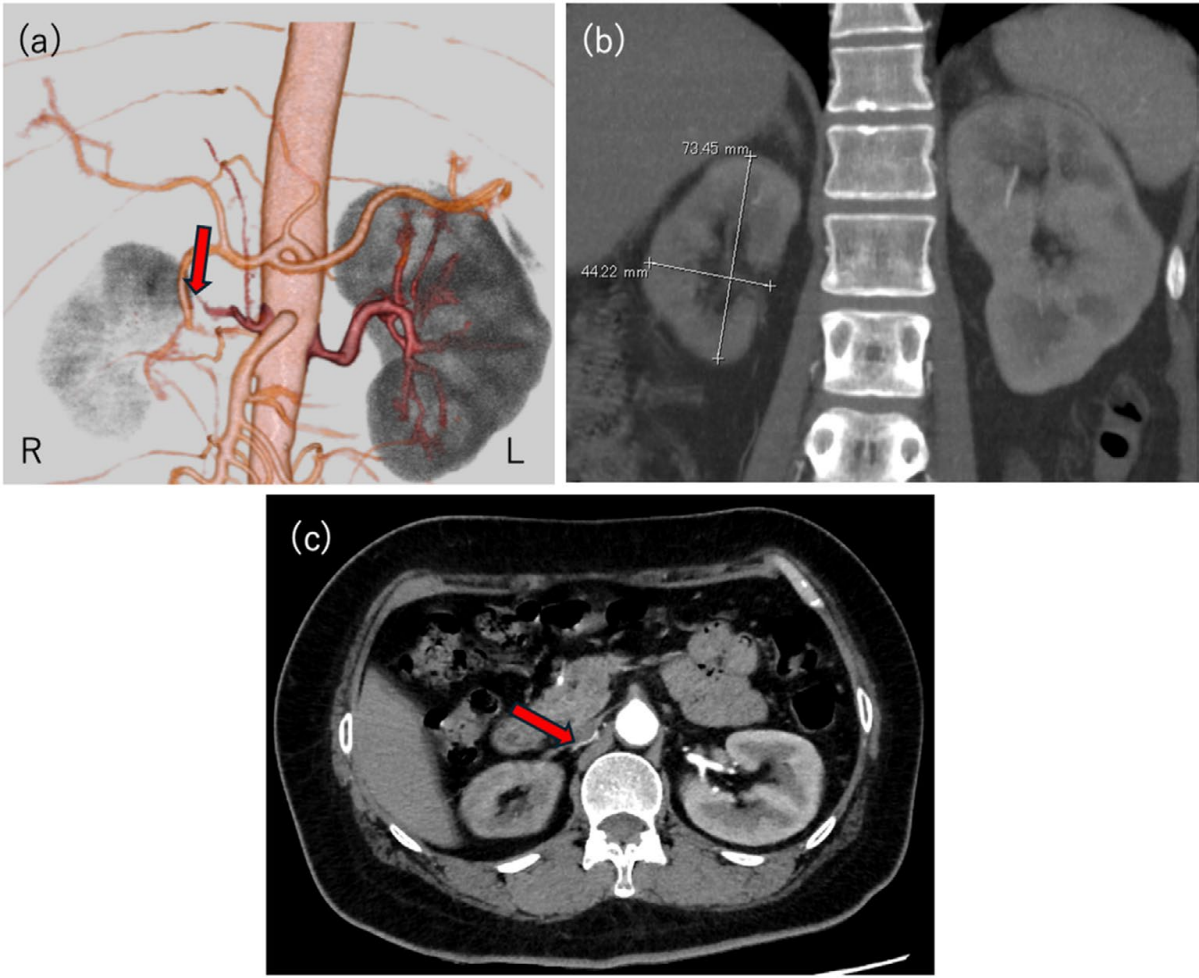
(a) 3D reconstruction of computed tomography (CT) images. Stenosis is observed in the right renal artery (arrow). (b) Contrast‐enhanced CT (coronal section). Right kidney atrophy of 7.3 cm by 4.4 cm. (c) Contrast‐enhanced CT (axial section). Stenosis is observed in the right renal artery (arrow).
